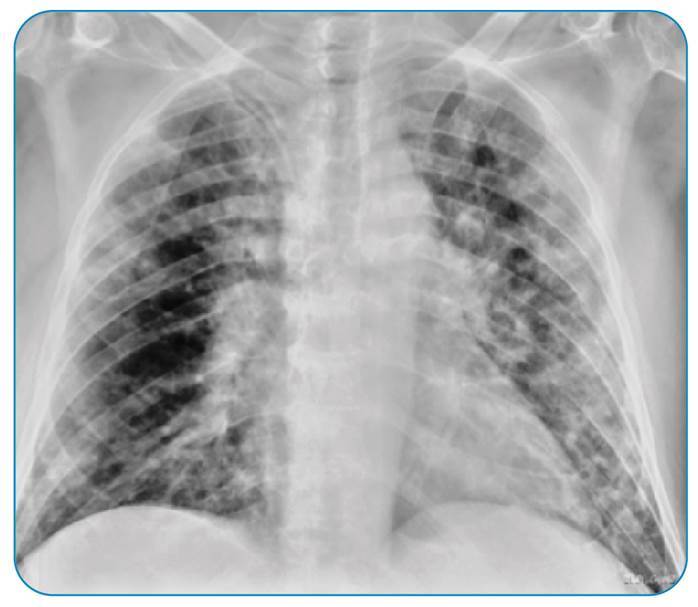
Chest radiograph at admission demonstrating diffuse bilateral interstitial infiltrates.
